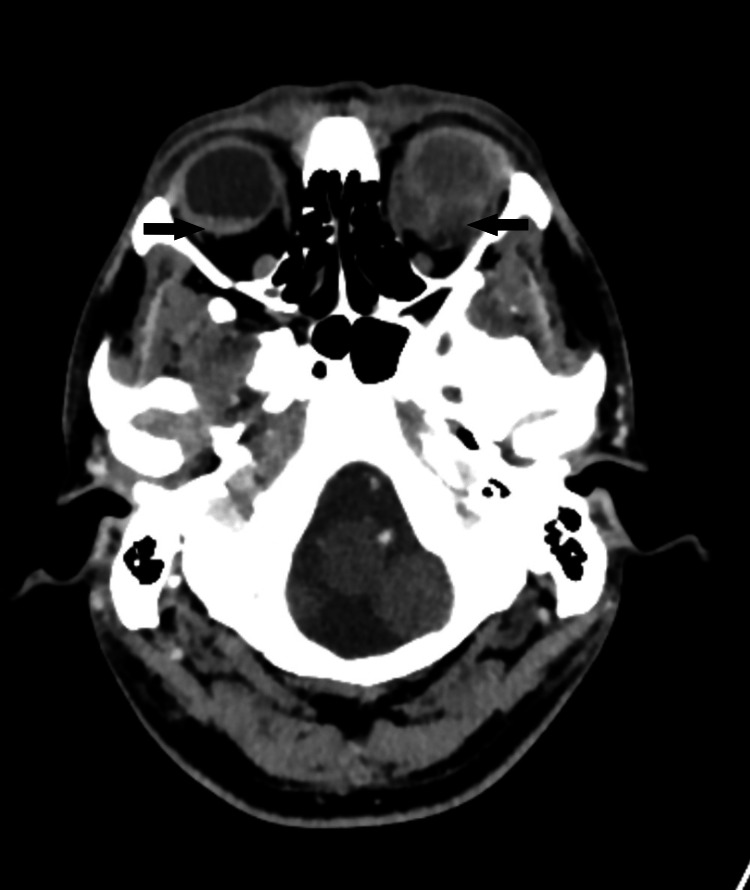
Contrast CT brain at the level of orbits demonstrates soft tissue masses involving bilateral globe (black arrows).
